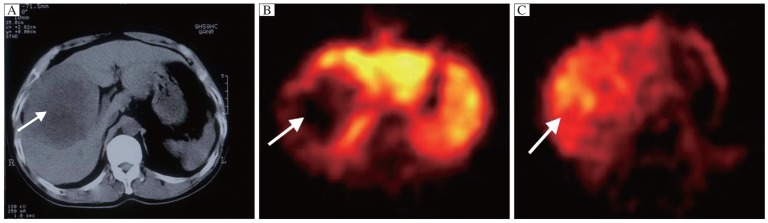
MRI and SPECT detection of hepatocellular carcinoma in the right liver. A: MRI image showed that the tumor was located in the posterior lobe of the right liver. B: ^99m^Tc-MIBI SPECT revealed that MIBI accumulated densely at tumor lesion. C: Significant MIBI uptake on ^99m^Tc-MIBI SPECT was noted in tumor lesion after intake of bromocriptine. Arrow indicates the site of tumor lesion.
